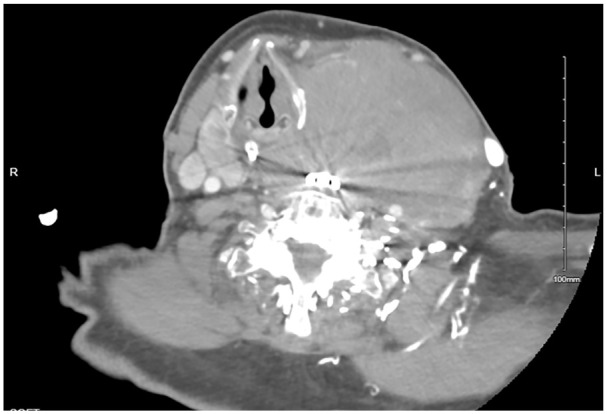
CT of the neck soft tissue with contrast: additional cystic nodule within the right thyroid lobe measuring 1.4 × 1.2 cm.
